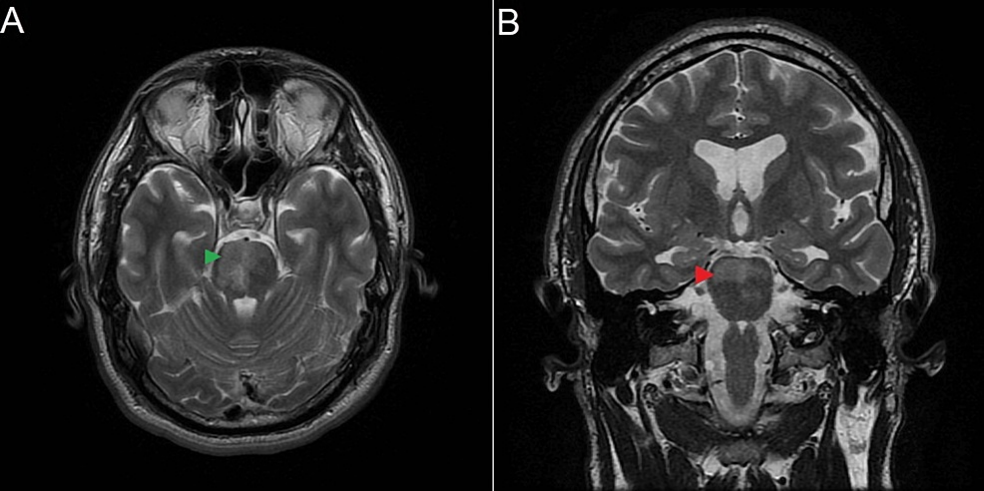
(A) Axial view brain MRI showing pontine infarction (green arrowhead). (B) Coronal view brain MRI showing pontine infarction (red arrowhead). Both images show pontine infarction. Brain MRI in axial and coronal T2WI shows high signal intensity involving the bilateral anteromedial aspects of the pons more prominent on the right side. MRI: magnetic resonance imaging; T2WI: T2-weighted image
